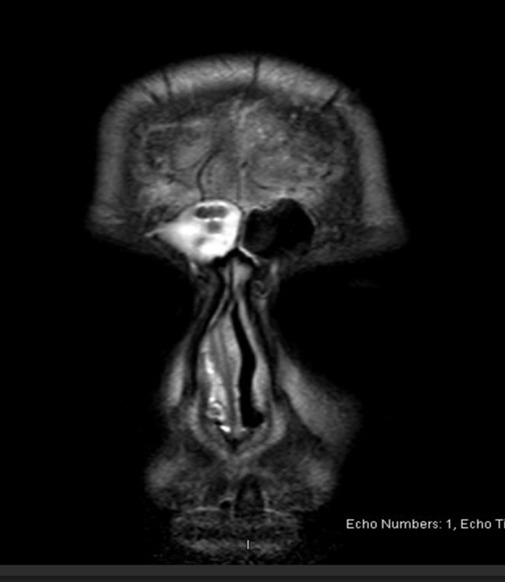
Brain magnetic resonance imaging with contrast of our patient. A) Demonstrates significant mucosal thickening of the maxillary sinus (arrow) consistent with recurrent sinusitis. B) Demonstrates return of right frontal osteomyelitis (arrow).
